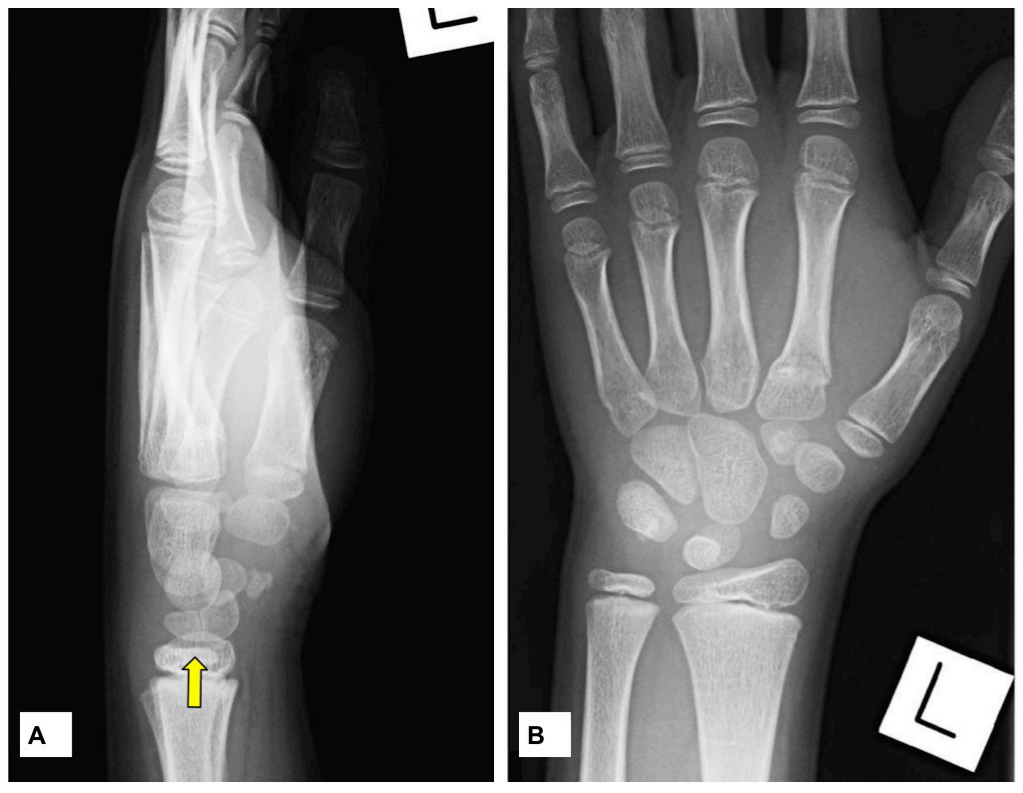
(A) Lateral and (B) posteroanterior radiographs of the patient's left hand. The bipartite lunate is clearly visible on the lateral radiograph (A; arrow). The margins of both lunate portions are smooth and well corticated.
